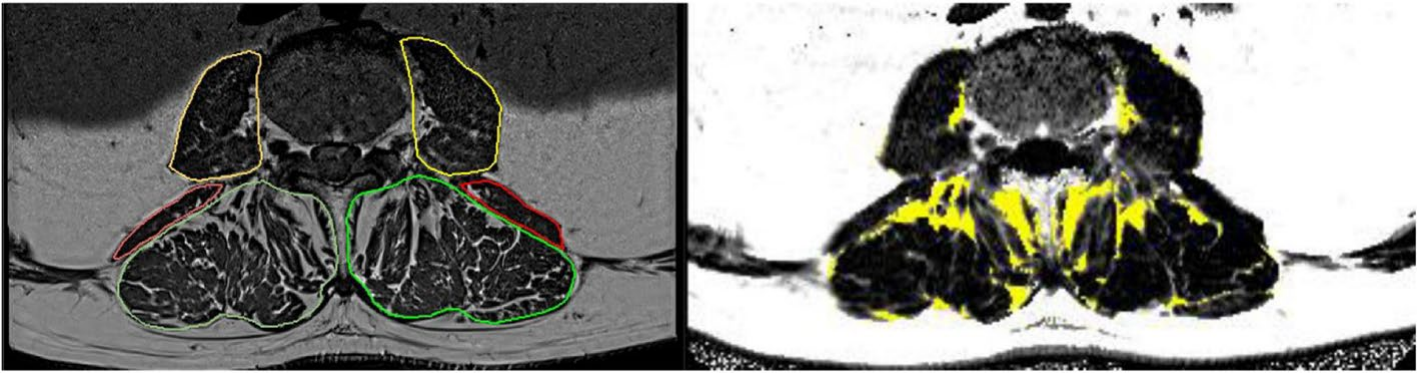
Left: Segmentation of T1-weighted MR images. The lines represent the fascial envelope of M. psoas (yellow), M. erector spinae (green) and M. quadratus lumborum (red). Right: IMAT within the fascial envelope in a Dixon MRI
